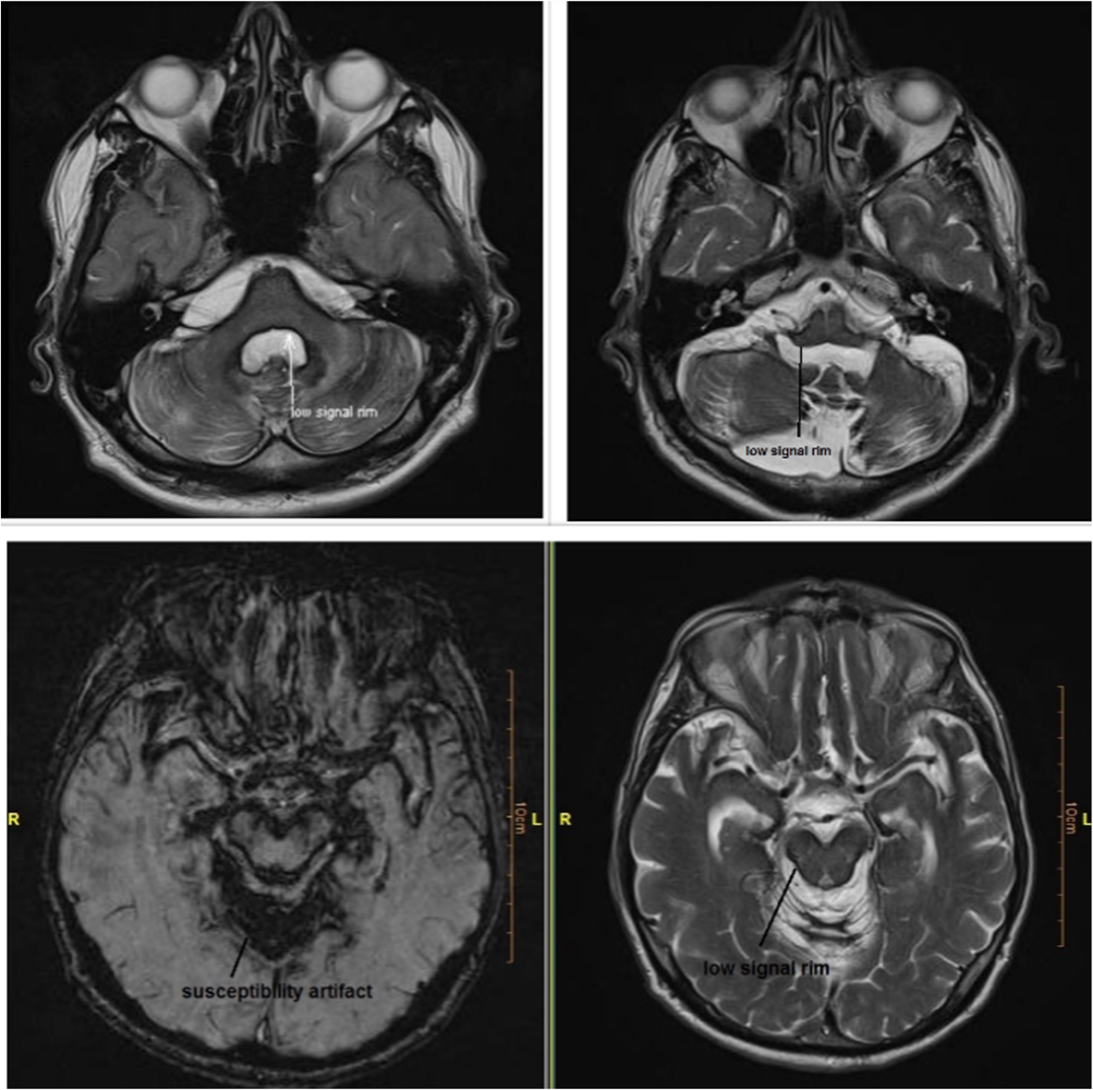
MRI brain images of the patient 1 showing features characteristic of superficial siderosis. 1. Low rim coating the surface of the brain and brain stem particularity cerebellum with obvious signal void intensity. 2. High signal intense rim in T1 sequences is coated brain stem and cerebellar vermis
